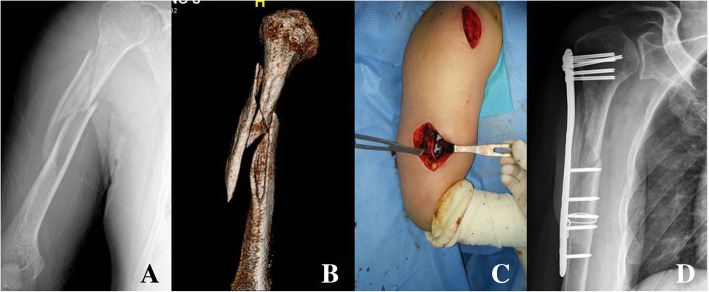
A 55 year-old male complained painful swelling of the upper arm after trauma. Conventional X-ray showed long spiral (Spiral wedge type, AO classification: B1) humerus shaft fracture with fracture extension to the proximal humerus (a, b). When using MIPO technique with long philos plate, the radial nerve was identified through the distal incision (c). Long humeral philos plate could fix this comminuted humeral shaft fracture adequately (d)
